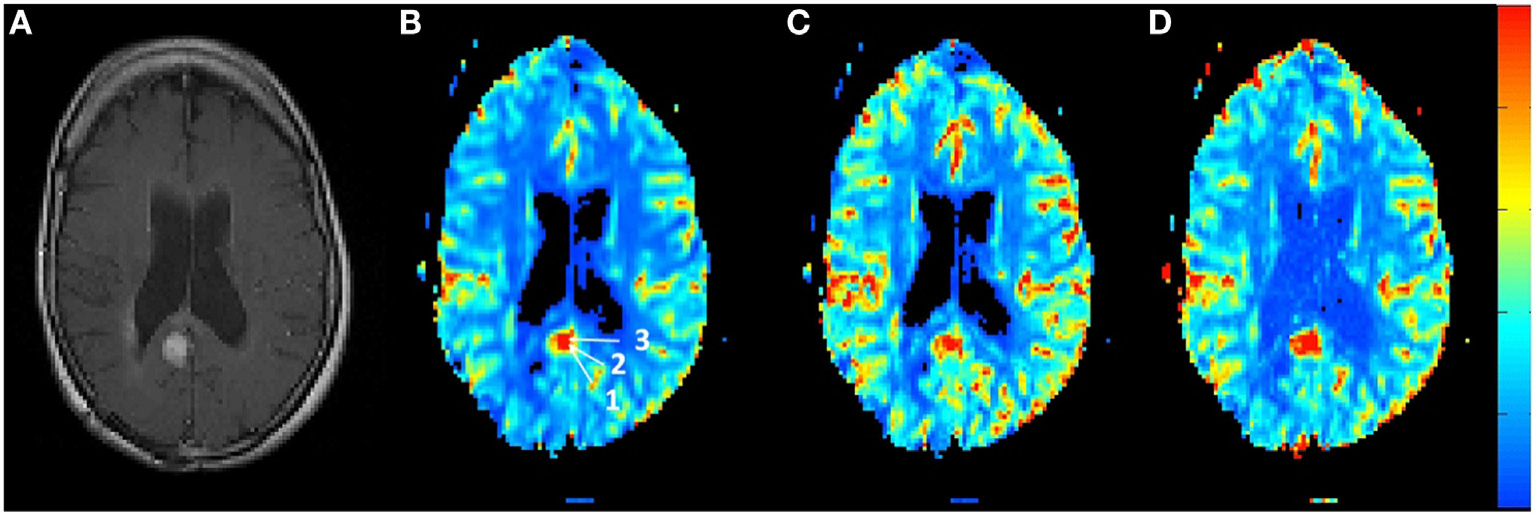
(A) T1-weighted post-contrast MRI showing the location of suspected tumor. The suspected enhanced region has a hot spot area in the (B) relative blood volume map as well as in the (C) relative cerebral blood flow map determined by the peak of the first-pass tissue residue function as well from the (D) second-pass tissue residue function.
